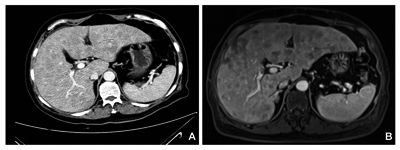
Abdominal computed tomography (A) and T1-weighted magnetic resonance imaging (B), revealing multiple occupying nodules in liver. Neither splenomegaly nor abdominal lymphadenopathy was observed.
